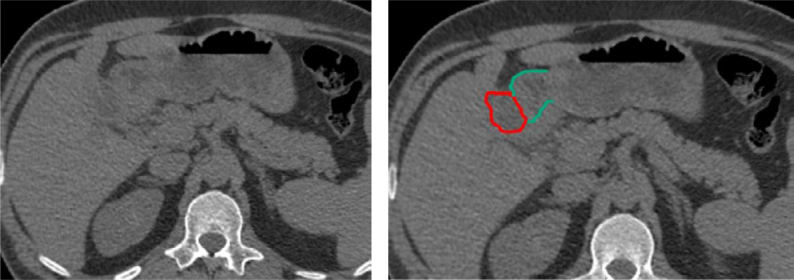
Abdominal computed tomography scans (without intravenous contrast) showing thickening of the duodenal wall and gallbladder, with a probable fistulous tract (left and right).
